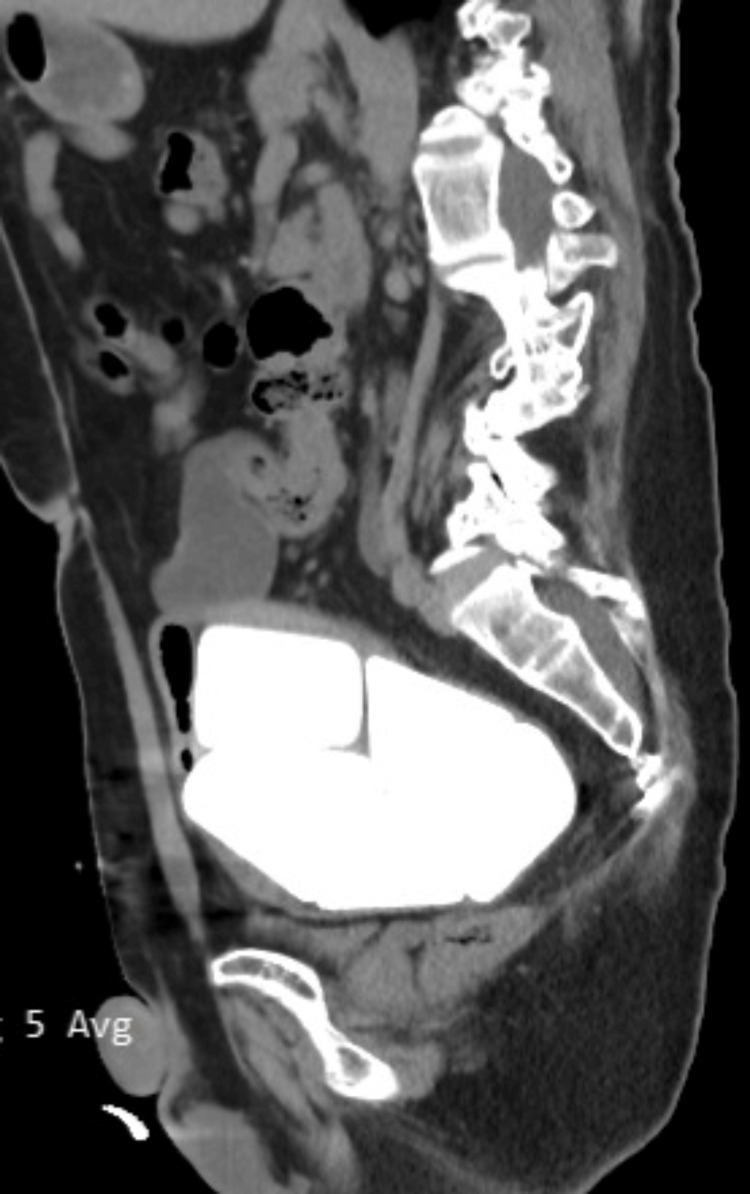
A sagittal CT image of bladder stones
